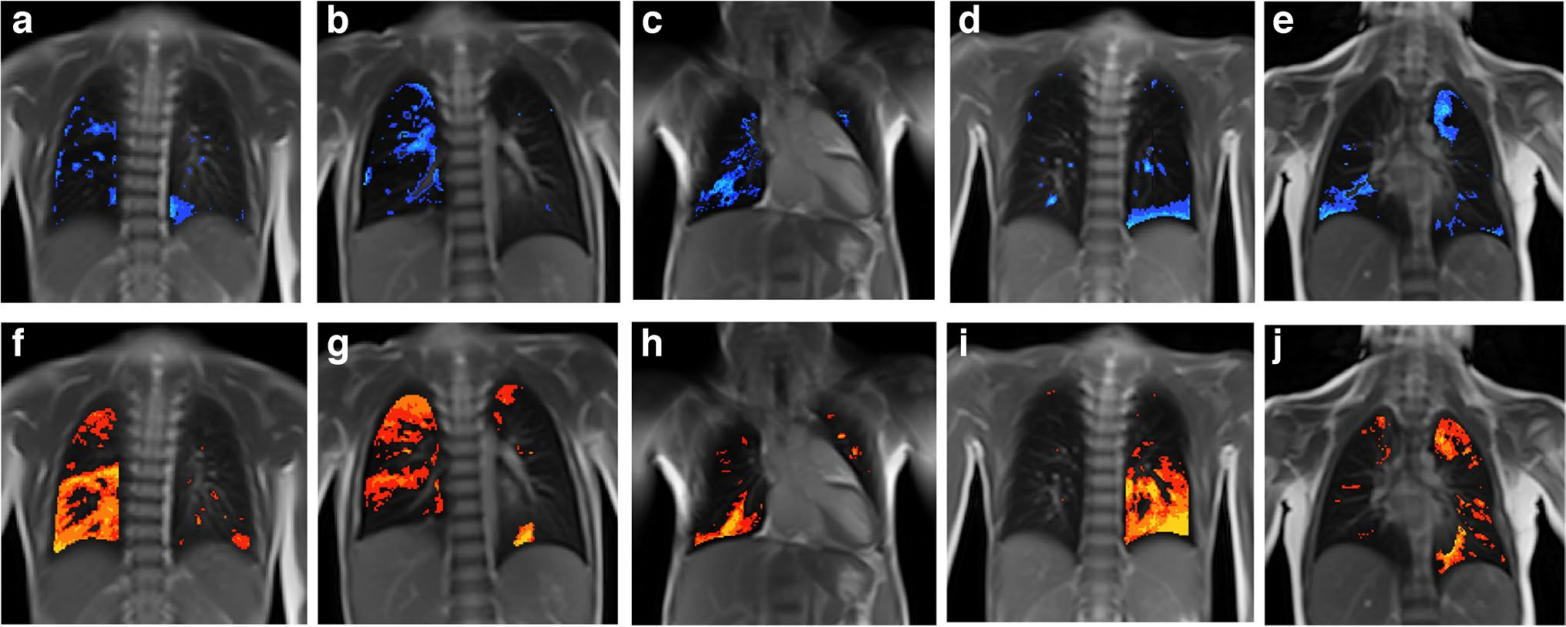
a-j Coronal functional magnetic resonance imaging maps with fractional ventilation defect maps in the upper row and perfusion defect maps in the lower row (brighter blue and orange areas represent reduced function). Case 1 (a, f) 7-year-old girl, case 2 (b, g) 5-year-old boy, case 3 (c, h) 10-year-old girl. Cases 1-3 after lobectomy of the right lower lobe. Case 4 (d, i) 6-year-old boy after lobectomy of the left lower lobe. Case 5 (e, j) 6-year-old girl after resection of left Seg I-III
